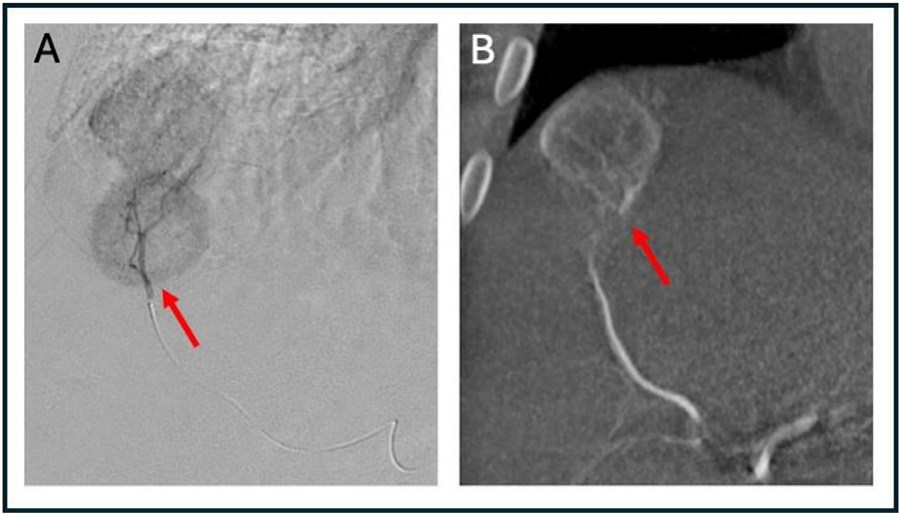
A selective hepatic arteriogram (A) demonstrates arterial supply to the segment 7 metastatic lesion subjacent to the hepatic capsule (red arrow). A Cone beam CT (B) confirms selective perfusion of the segment 7 lesion (red arrow). Minimal arterial enhancement is identified within the background parenchyma suggesting no variant anatomy or anomalous arterial supply. Following arterial mapping, this lesion was treated with Y90 TheraSpheres.
